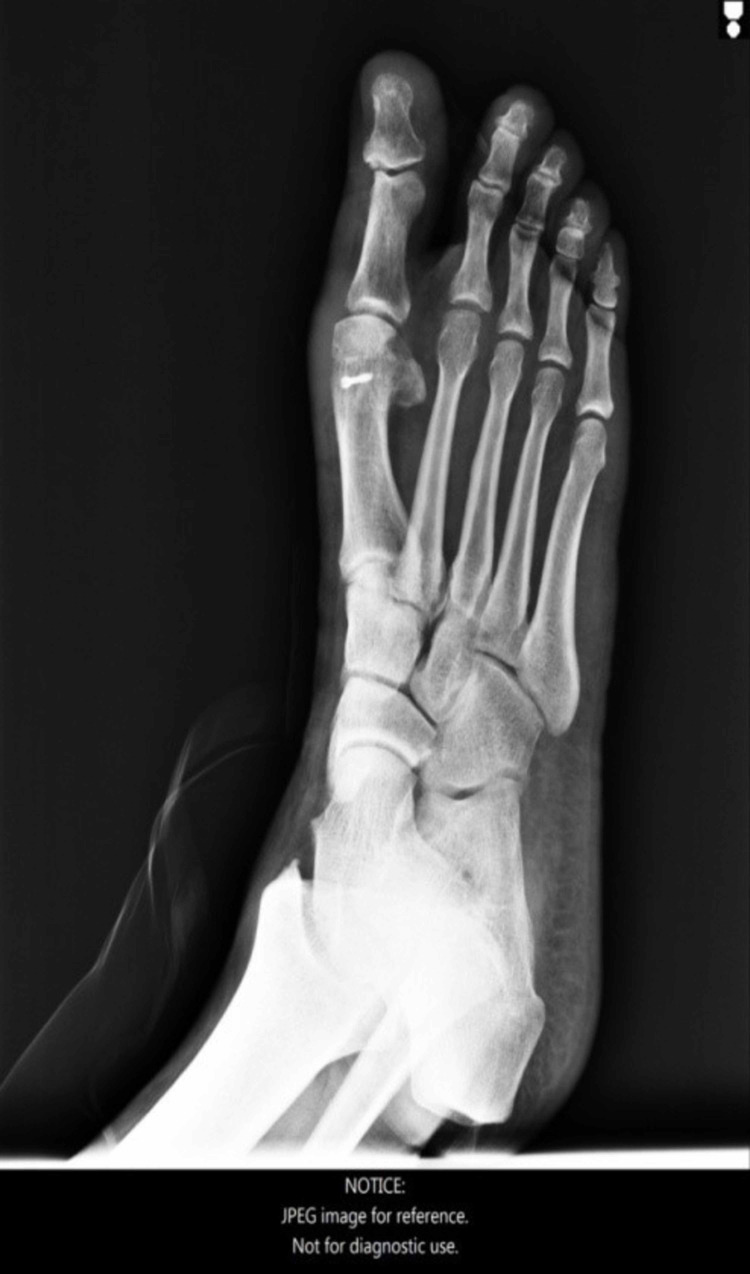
Foot X-ray, lateral view, 27 months post-operatively.
